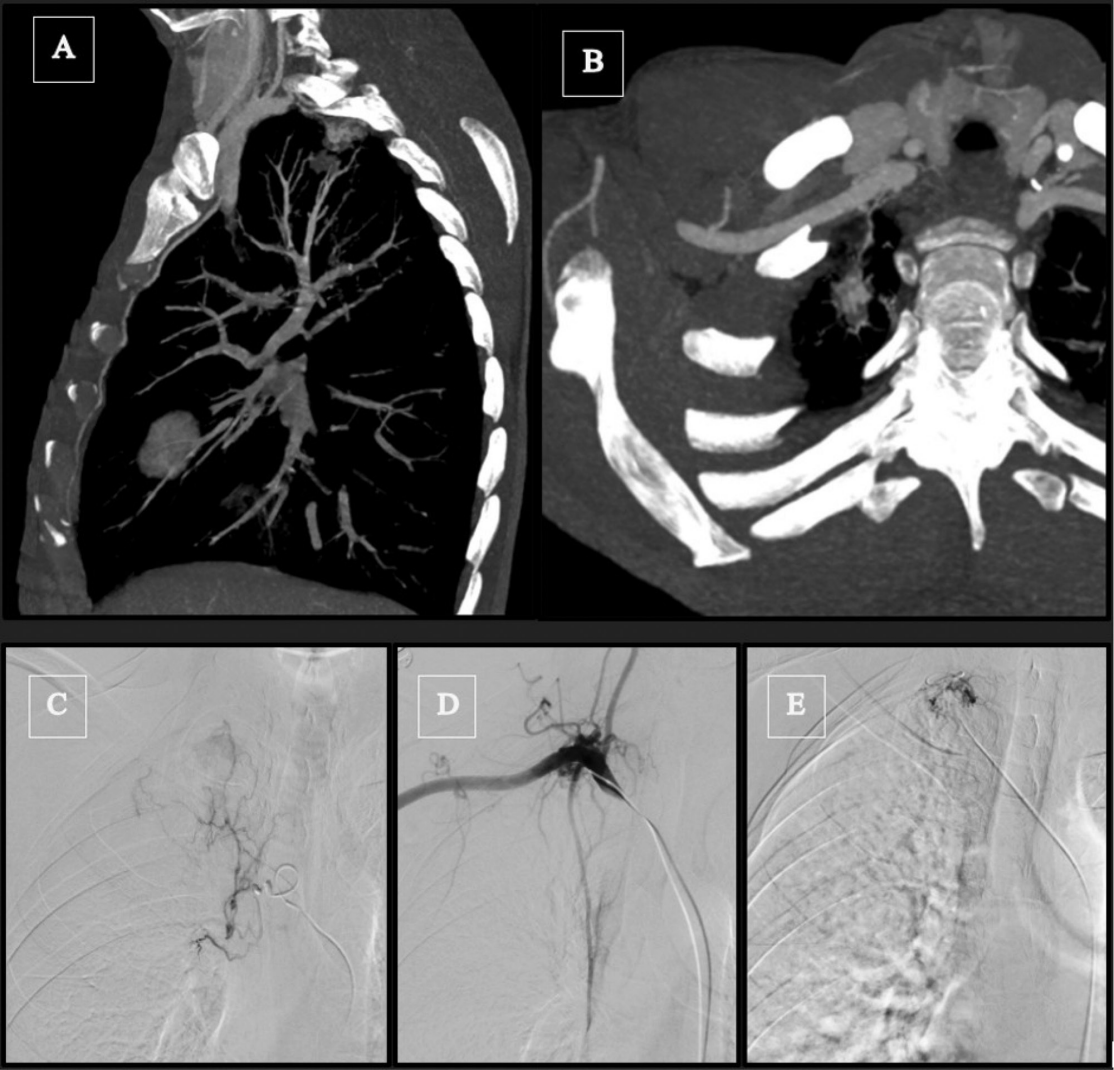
Forty-year-old patient with a right upper lobe arteriovenous malformation (AVM) or vascular degeneration mimicking AVM post-tuberculosis sequelae. (A, B) Angiography CT sagittal maximum intensity projections (MIP) and volume rendering technique (VRT) showing a vascular nidus located in the right upper lobe with the participation of a NBSA coming from the subclavian artery. (C) Angiography of the right bronchial artery shows blushing at the lesion site. (D, E) Angiographic catheterization and embolization of the NBSA coming from the subclavian artery.
